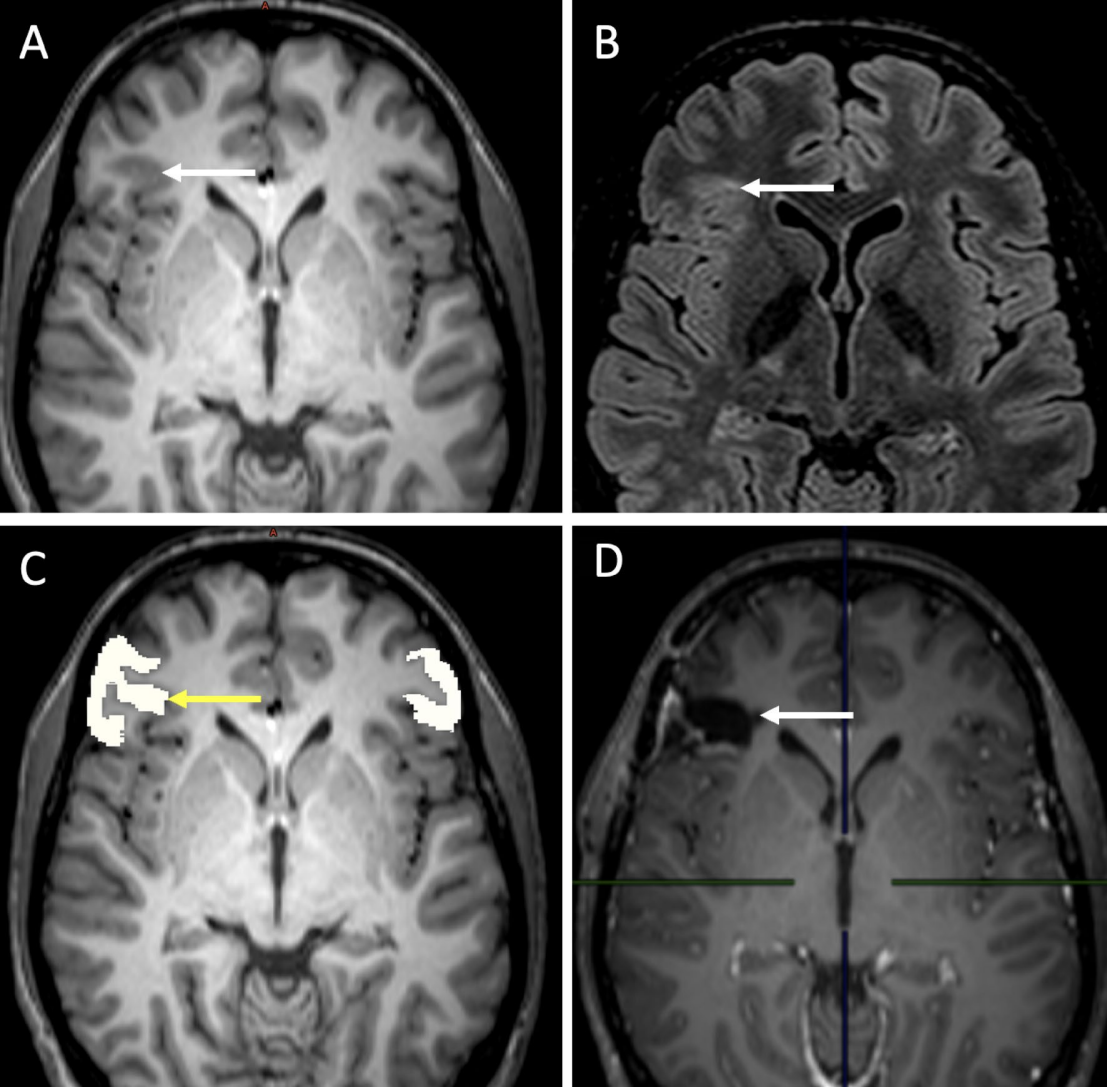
Example of a 18-year old patient with surgically confirmed FCDII of the right inferior triangular gyrus; MRI was considered abnormal by the senior radiologist. (A) Presurgical T1-weighted imaging: the white arrow shows focal thickening of the cerebral cortex at the level of the right inferior triangular gyrus. (B) Presurgical FLAIR sequence: the white arrow shows a subcortical region with FLAIR signal hyperintensity at the level of the dysplastic zone. (C) AssemblyNet segmentation of the right inferior triangular gyri, the yellow arrow shows the right sided region. (D) Postsurgical T1-weighted scan: postoperative cavity (white arrow).
